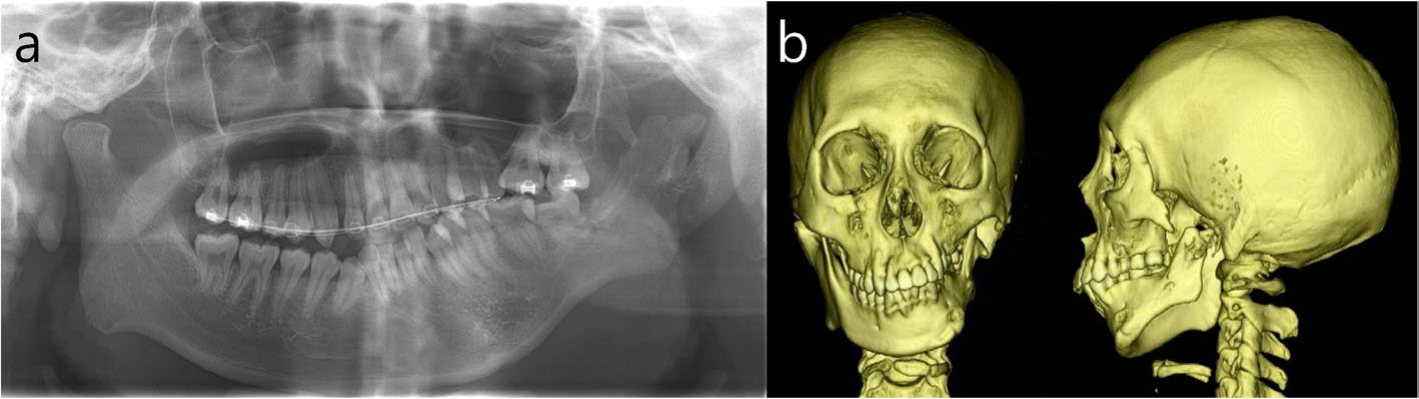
Radiographs on initial visit. a Panoramic view. b frontal and lateral views of 3D CT
